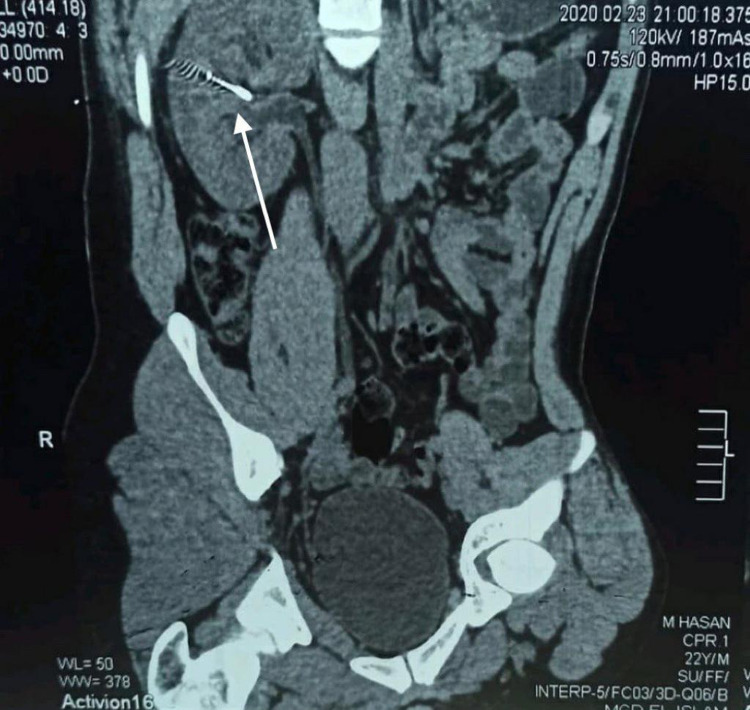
Abdominal CT without contrast shows a 2-inch nail within the right kidney.
